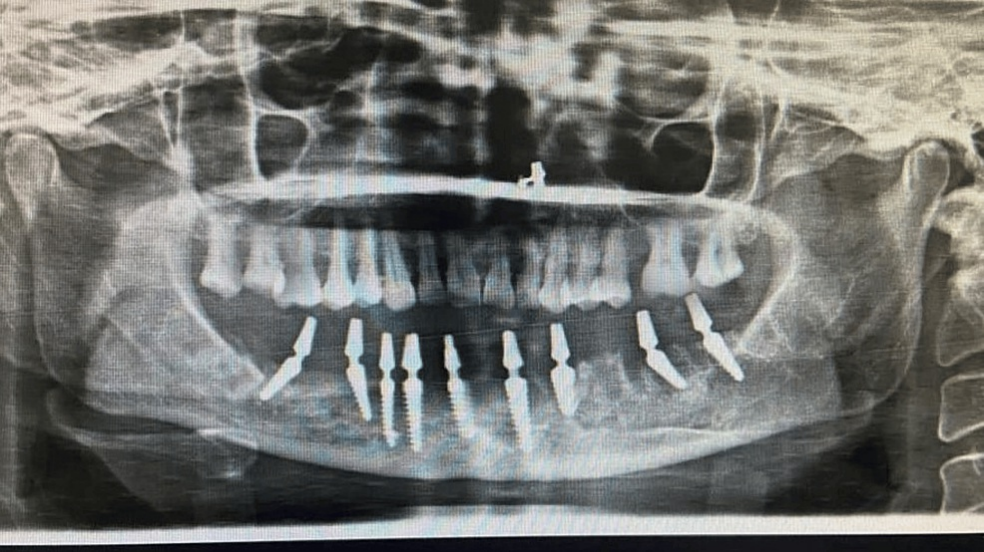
Post-operative radiograph after the placement of basal implants
